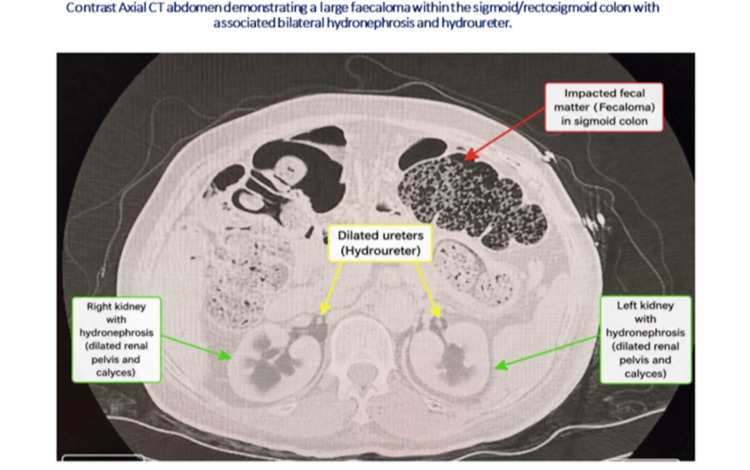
Contrast-enhanced CT of the abdomen demonstrating fecaloma with bilateral hydronephrosis and hydroureter. Axial contrast-enhanced CT of the abdomen demonstrating a fecaloma (red arrow) within the sigmoid colon, consistent with fecal impaction. There is associated bilateral hydronephrosis (green arrows). The ureters appear prominent (yellow arrow), in keeping with hydroureter measuring approximately 0.9 cm on the right and 0.8 cm on the left, in the context of bilateral hydronephrosis. Overall findings are consistent with stercoral colitis causing extrinsic compression of the distal ureters and resultant obstructive uropathy.
